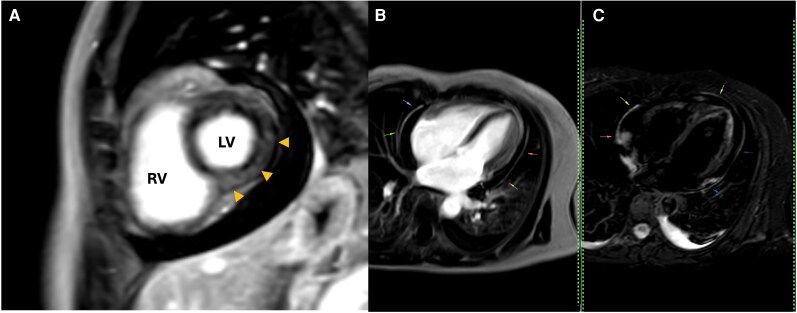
Cardiovascular magnetic resonance images demonstrating. *(A)* Subepicardial non-ischaemic late gadolinium enhancement in the inferior and inferolateral basal and mid left ventricular segments (arrows), consistent with myocardial inflammatory involvement. *(B and C)* Four-chamber view image in late post-contrast T1-weighted and T2-weighted showing late gadolinium enhancement oedema at the level of the pericardium.
